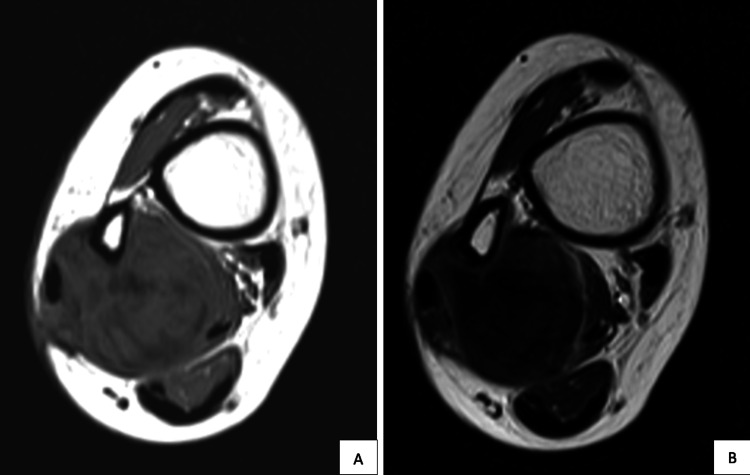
Pre-operative MRI image in axial cut showing the mass located between the flexor hallucis longus, tibialis posterior, and distal soleus muscle in T1- (A) and T2-weighted images (B) MRI, magnetic resonance imaging
